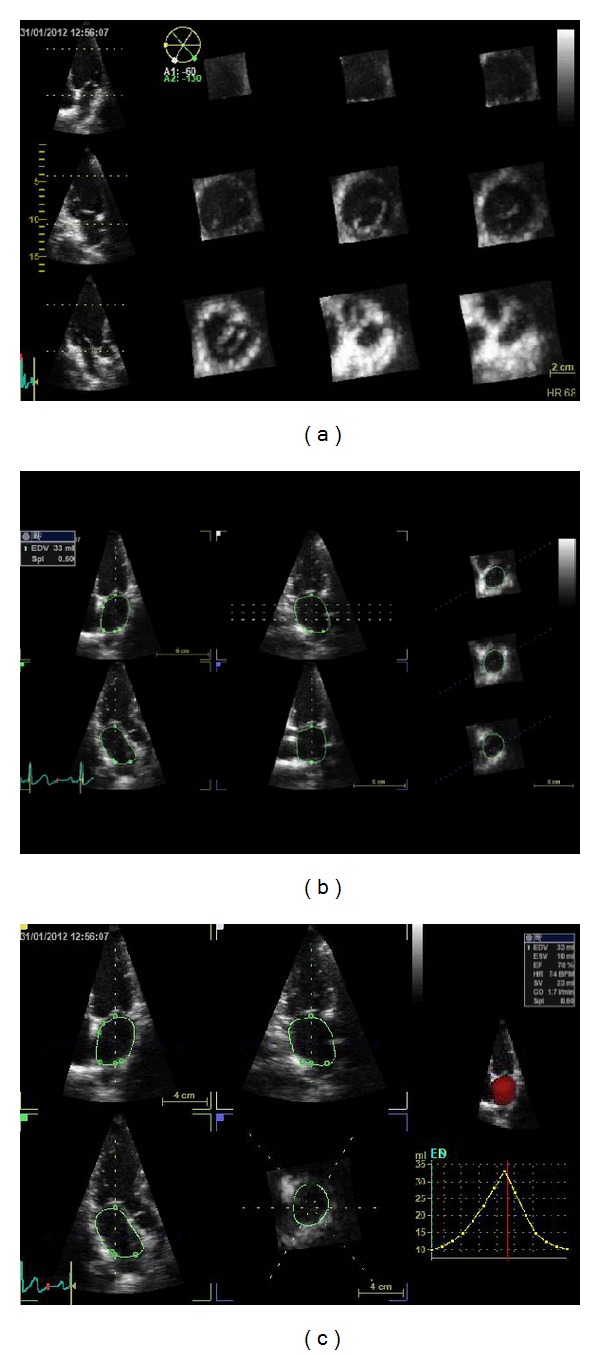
Left atrial volumes by LVQ Auto 4D echocardiography. (a) Left atrial full-volume data sets are acquired. (b) After manually initializing one point to identify the mitral valve plane and another point for the centre of LA roof, the program automatically identifies the endocardial surface both in end-systole and in end-diastole. (c) Atrial maximum (max) and minimum (min) volumes are obtained and displayed in curve, in numeric values, and in 3D image (in red).
